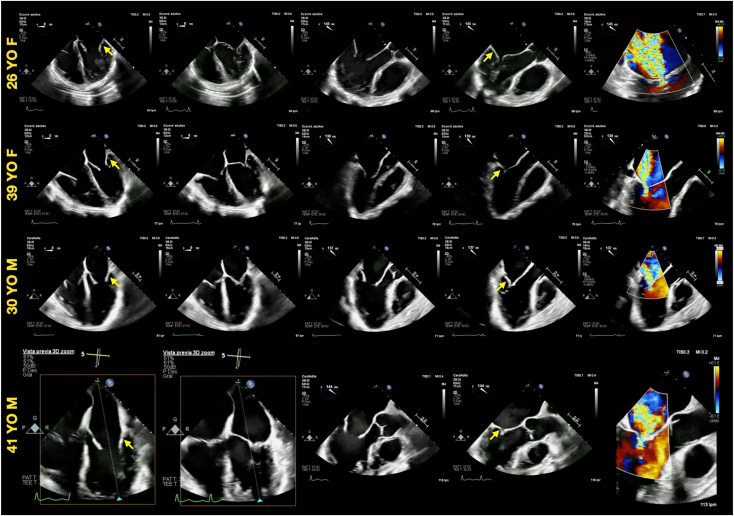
Two-dimensional morphologic assessment of the mitral valve two-dimensional TEE, midesophageal 4-chamber (0°) and long-axis (130°-145°) views, in both diastole and systole (with color-flow Doppler), demonstrates diffuse thickening of the MV leaflets—most pronounced in the posterior leaflet—and restricted leaflet closure (*yellow arrows*). All 4 patients are displayed to demonstrate the similar valve pathology with predominant involvement of the posterior leaflet and an eccentric MR jet directed inferolaterally.
